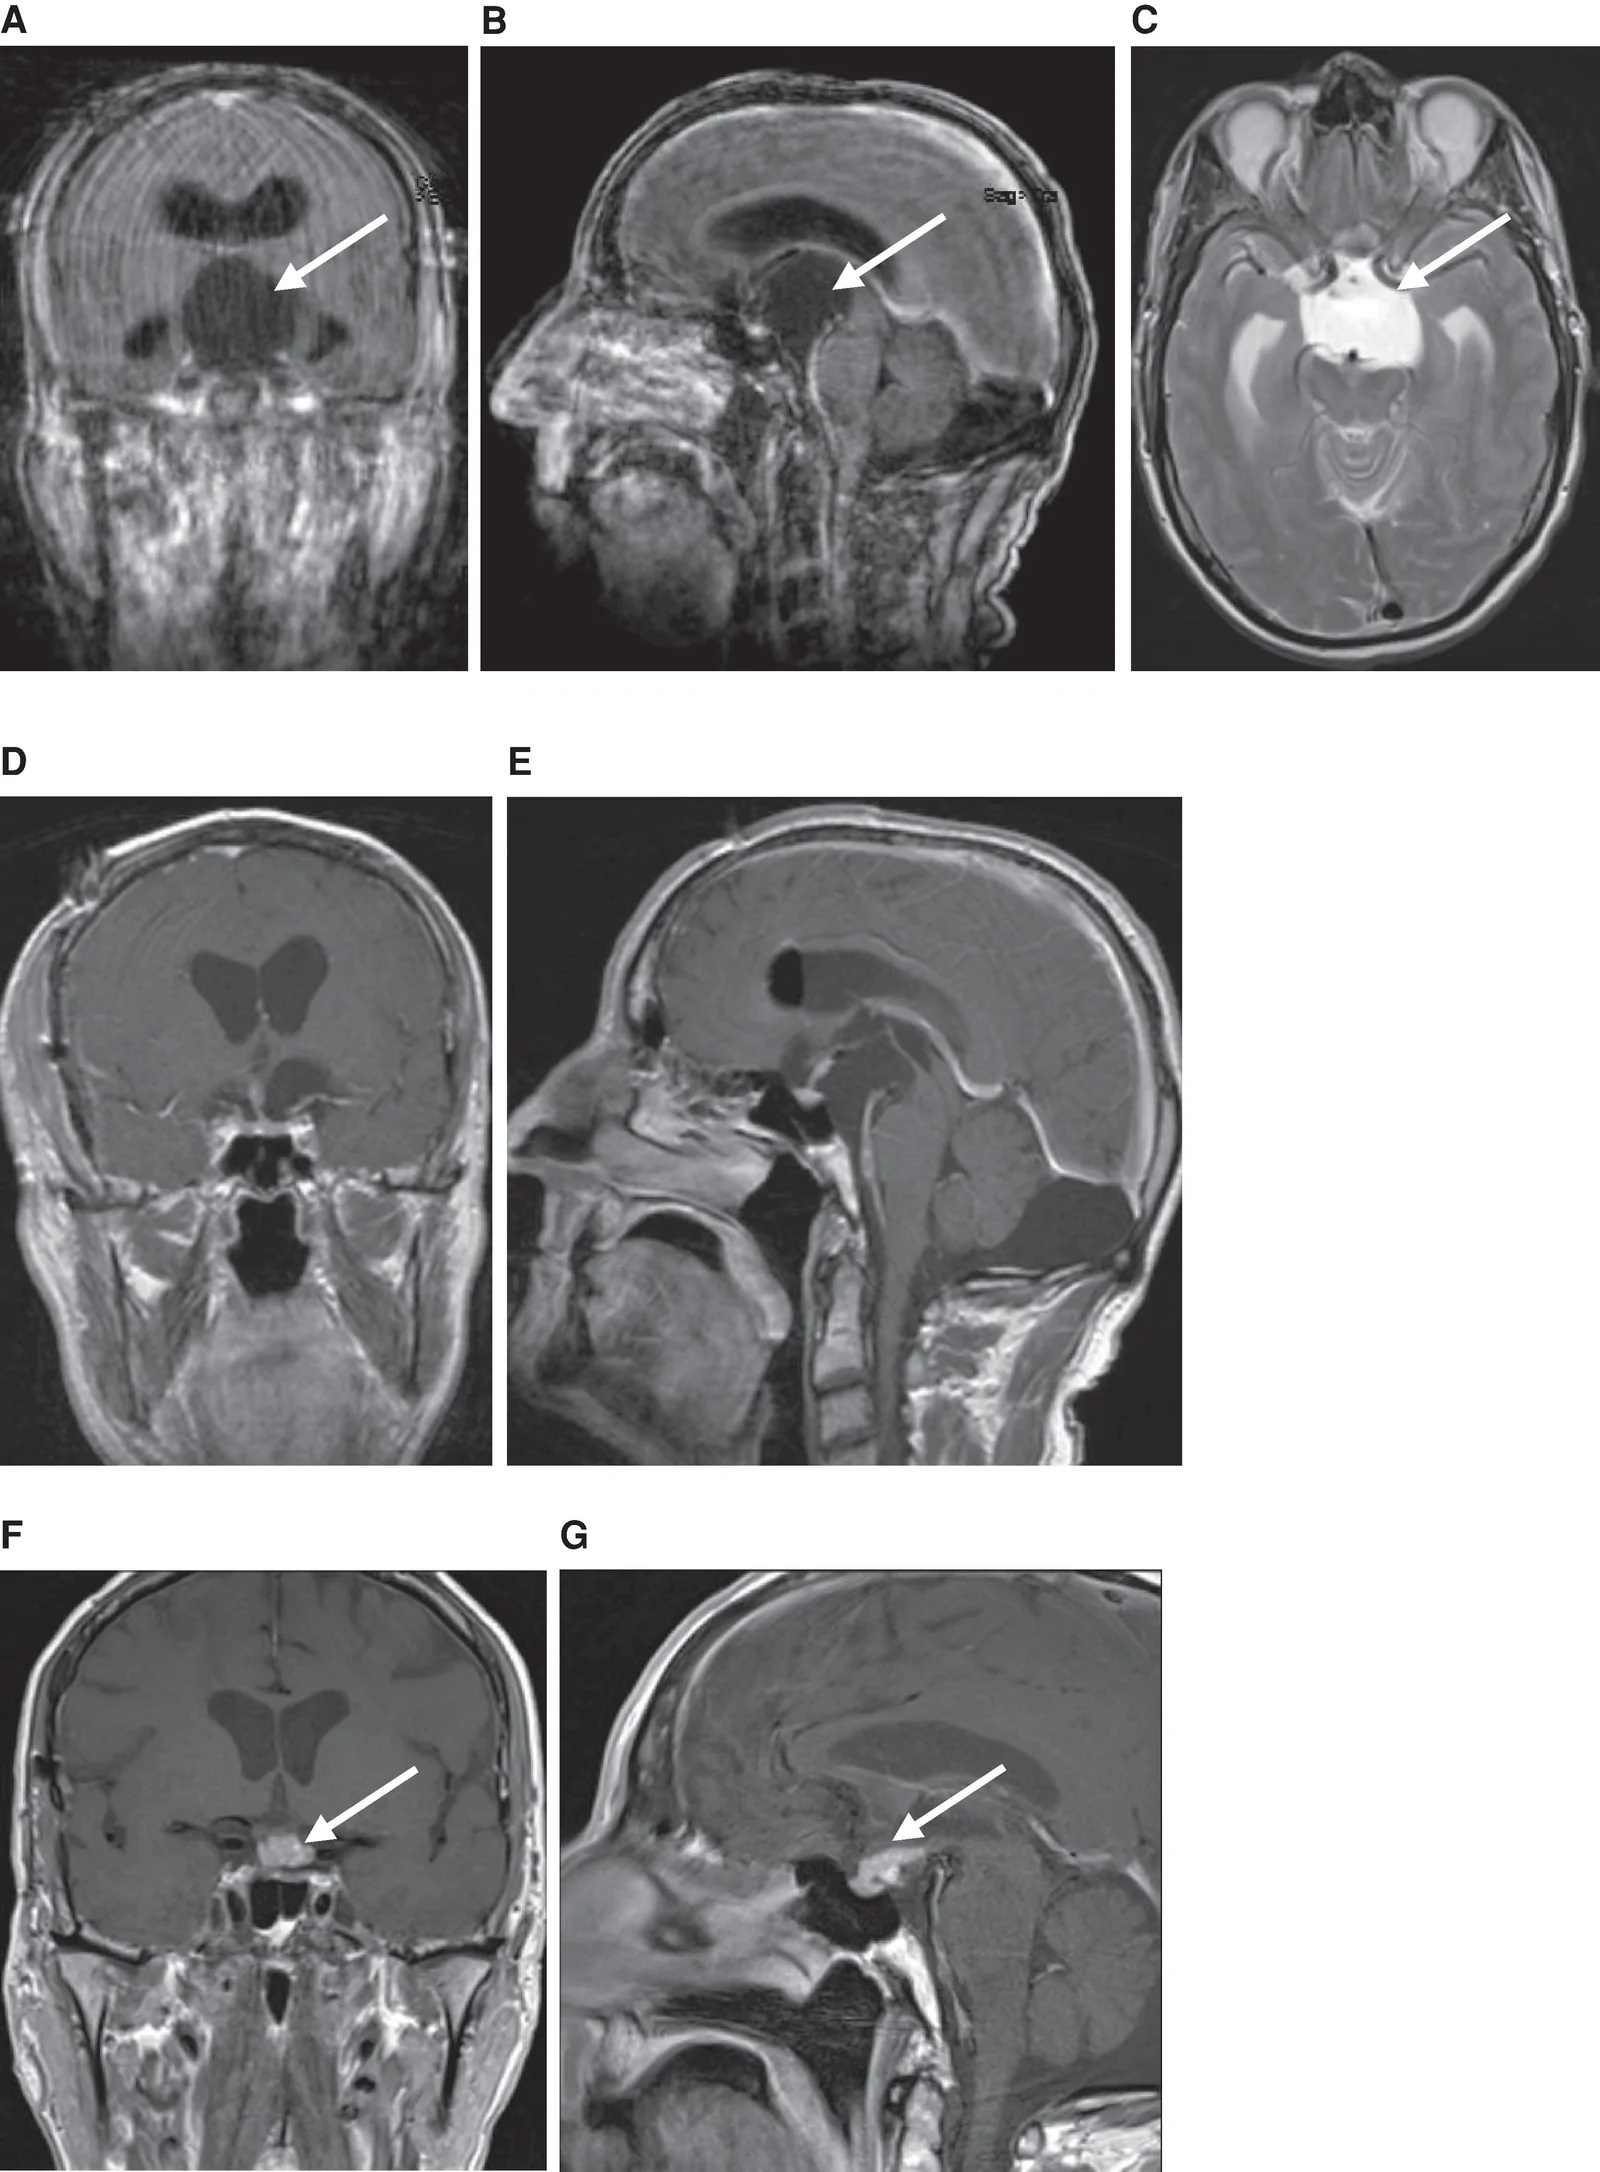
Magnetic resonance imaging (MRI) scans. A-C) At presentation in 2015: coronal post-contrast T1 (A), sagittal post-contrast T1 (B), and axial T2 (C) images demonstrating a large predominantly cystic suprasellar/interpeduncular lesion (white arrows) causing obstructive hydrocephalus with ventricular dilatation and periventricular edema. D-E) Postoperative imaging in 2015: coronal (D) and sagittal (E) post-contrast T1 images following right frontal craniotomy and cyst fenestration, demonstrating marked decompression of the cystic lesion and improvement in hydrocephalus. F-G) Recurrence in 2021: coronal (F) and sagittal (G) post-contrast T1 images demonstrating development of a new enhancing mixed solid-cystic suprasellar lesion adjacent to the pituitary stalk and optic chiasm (white arrows), later confirmed as papillary craniopharyngioma.
